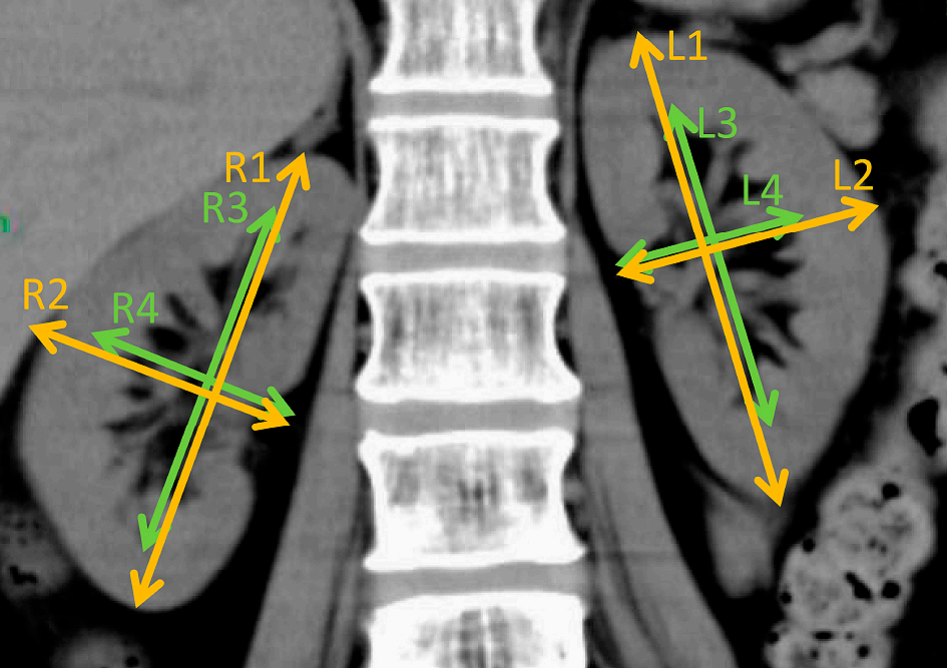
Coronal computed tomography image used to obtain total kidney volume. The maximum length of the kidney (R1-2, L1-2) and renal pelvis (R3-4, R3-4) was measured in the longitudinal and transverse axes. Using these lengths, the kidney volume was calculated by the equation described in the Methods section.
